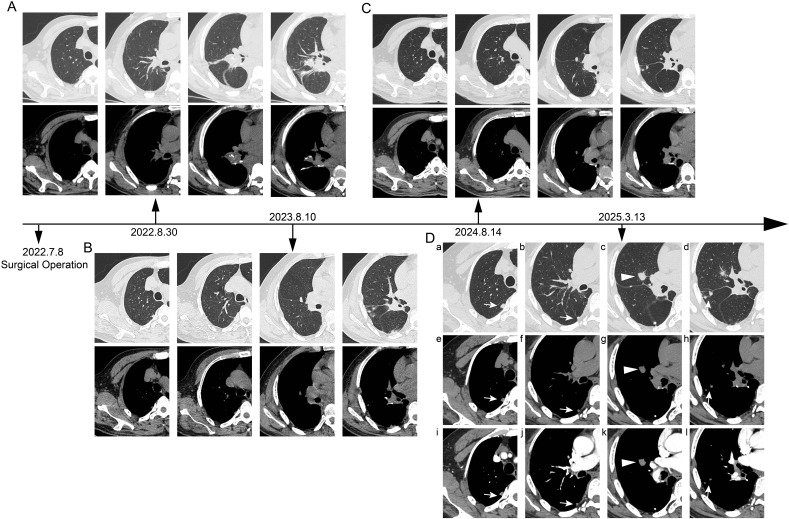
Timeline of postoperative chest CT follow-up. (A) Postoperative day 53 (August 30, 2022): Chest computed tomography (CT) scan revealed local atelectasis at the surgical site, with no new subpleural lesions observed. (B) 1 year postoperatively (August 10, 2023): Follow-up chest CT demonstrated a solid nodule (approximately 16×13 mm) in the lateral segment of the right middle lobe, along with additional subpleural solid nodules. (C) 2 years postoperatively (August 14, 2024): Follow-up chest CT showed no significant interval change in the nodule within the lateral segment of the right middle lobe or the subpleural nodules compared to the previous year. (D) Preoperative contrast-enhanced chest CT. On lung window (a-d) and mediastinal window (e-h) settings, several round nodules (open arrows and arrowheads) are observed in the subpleural region of the right upper lobe and the right middle lobe (c, g). The nodule in the right middle lobe exhibits short spiculations and pleural indentation. (i-l) The nodules in the subpleural area of the right upper lobe and the right middle lobe demonstrate contrast enhancement.
